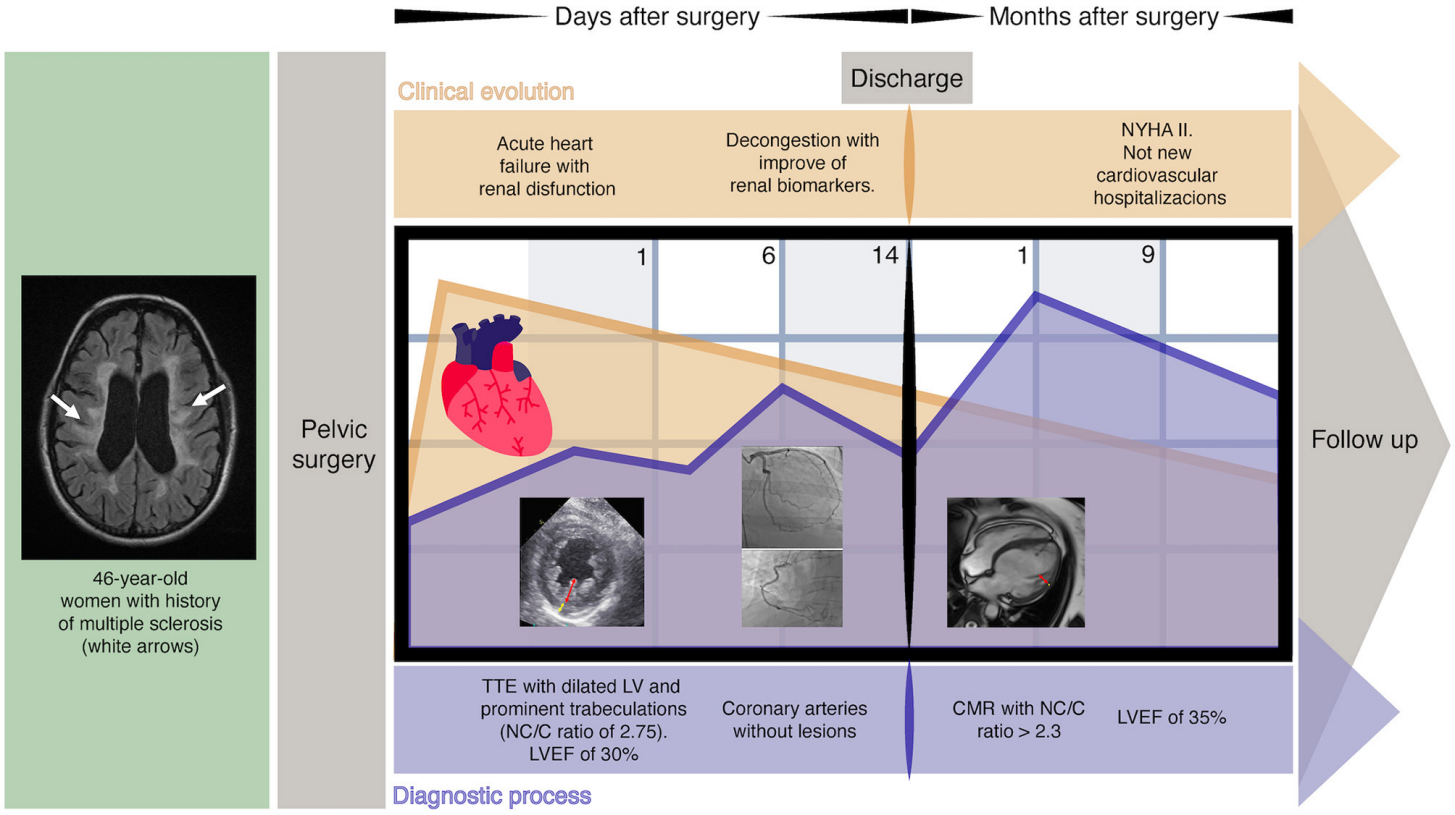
The timeline of events. CMR, cardiac magnetic resonance; LV, left ventricle; LVEF, left ventricular ejection fraction; NC/C, non-compacted to compacted; NYHA, New York Heart Association; TTE, transthoracic echocardiogram.
